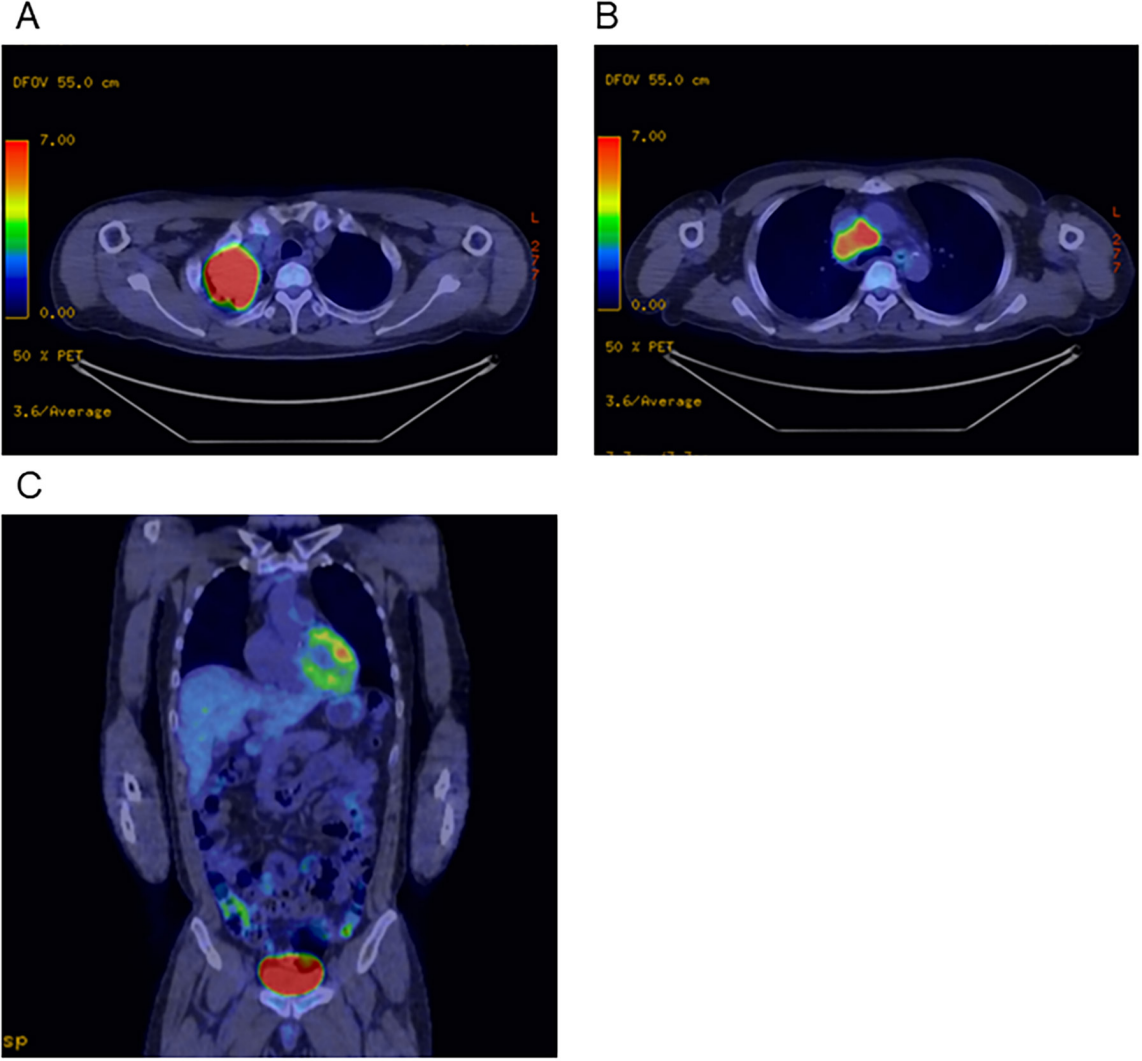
(A, B) Chest PET‐CT images revealing increased FDG uptake in the mass in the right upper lobe and the mediastinal lymph nodes. (C) PET‐CT did not reveal any FDG uptake in the abdomen. FDG, fluorodeoxyglucose; PET‐CT, positron emission tomography/computed tomography.
